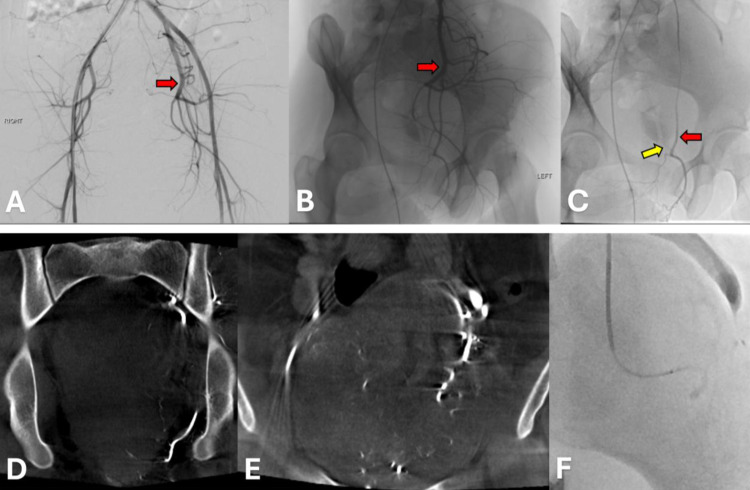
Pelvic angiography and cone beam CTs with embolization of a hypovascular AAM through the left uterine artery. A: A 5 Fr Contra flush catheter was advanced into the upper abdominal aorta (not pictured) via the right common femoral artery. Nonselective pelvic angiography in the AP projection revealed the mass had a dominant arterial supply from the left internal iliac artery (red arrow). B: A 5 Fr RBT catheter was used to select the left internal iliac artery (red arrow). Selective angiography in the right anterior oblique projection redemonstrated the findings in A. C: A 2.8 Fr Progreat microcatheter was advanced into the left uterine artery (red arrow). Angiography confirmed that the mass received most of its blood supply from this location. However, the mass itself was relatively hypovascular, similar to the contrast-enhanced pelvic MRI. Minimal arterial supply was seen in the bladder. D & E: Coronal intraoperative cone beam CTs confirmed a few distal branches of the left uterine artery predominantly supplied the mass. Incidentally, the patient was found to have two left ureters and one right ureter. F: Embolization of the left uterine artery was performed with 500-700 micrometer Embospheres. Post-embolization angiography revealed stasis of arterial flow to the mass.
